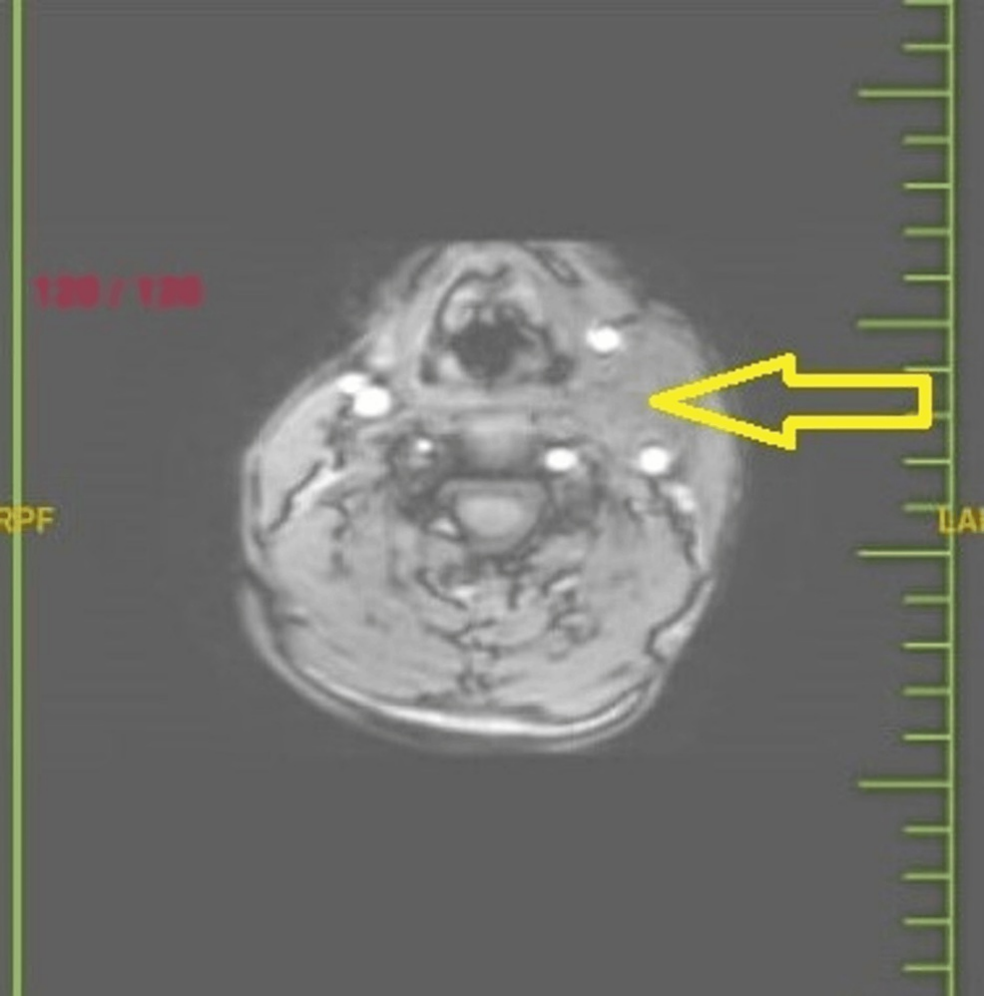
MRI brain showed a soft tissue mass lesion in the carotid space causing a mass effect suggestive of a carotid body tumor.
